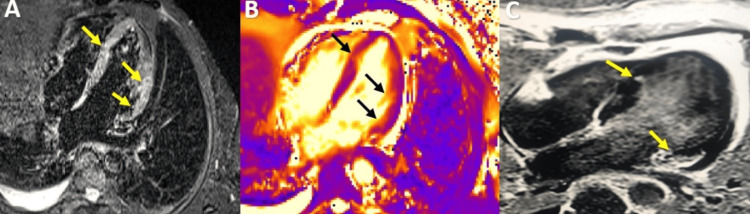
CMR examination of a 41-year-old male patient with toxocariasis with cardiac involvement CMR: Cardiac magnetic resonance Horizontal long axis (HLA) views: T2-weighted STIR image (A) and T2 mapping image (B) showing subendocardial oedema in the inferolateral wall and medium inferior septum (yellow arrows).  (C) Late gadolinium enhancement images show focal fibrosis at the level of basal inferolateral wall and medium inferior septum (yellow arrows).  Right pleural effusion is present. Image courtesy: Personal archive of Dr. Oana Popa. Patient consent was obtained.
